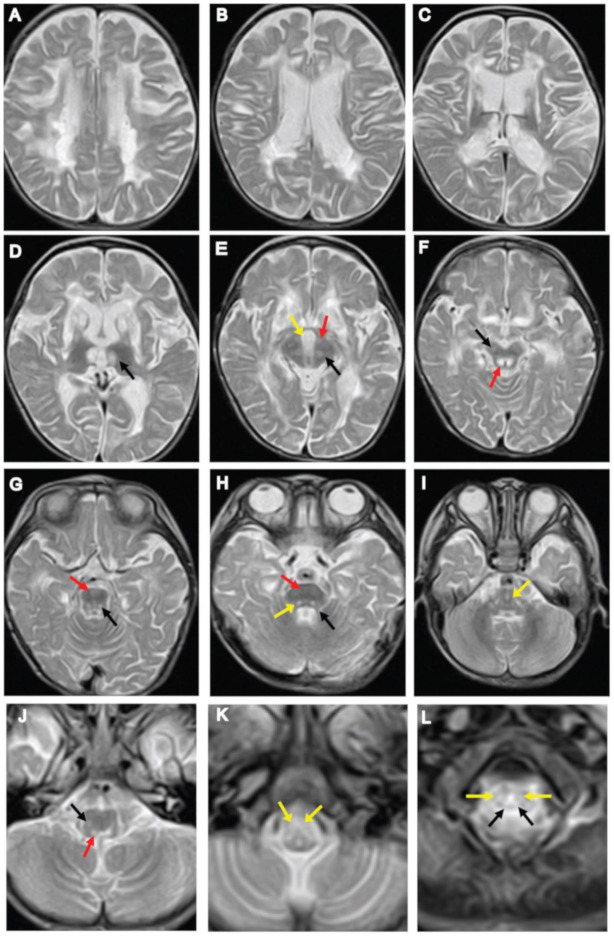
Neuroimaging findings at three years of age. (A–C) Axial T2-weighted images of brain MRI showing signal abnormalities in the periventricular white matter, putamen, caudate nucleus, splenium of the corpus callosum, and anterior and posterior limb of the internal capsule. (D) Symmetrical bilateral areas of increased signal intensity in the medial thalamic nucleus (black arrow). (E,F) Axial T2-weighted images of the midbrain: (E) interpeduncular cistern (yellow arrow), cerebral peduncle (red arrow), substantia nigra (black arrow); (F) substantia nigra (black arrow) and periaqueductal region (red arrow). (G–I) T2-weighted images of the pons: (G) pyramidal tract (red arrow), pontine tegmentum (black arrow); (H) superior cerebellar peduncle (black arrow), pyramidal tract (red arrow), pontine tegmentum (yellow arrow); (I) diffuse hyper-intensive lesions in the medial lemniscus (yellow arrow). (J,K) T2-weighted images of the medulla oblongata shows abnormal intensities in the medullary tegmentum, spinocerebellar tracts, and pyramids: (J) medullary tegmentum (red arrow), spinocerebellar tract (black arrow); (K) pyramids (yellow arrow). (L) T2-weighted images of the upper cervical spinal cord revealed abnormal intensity within the lateral corticospinal tracts (yellow arrows) and the dorsal columns (black arrows).
